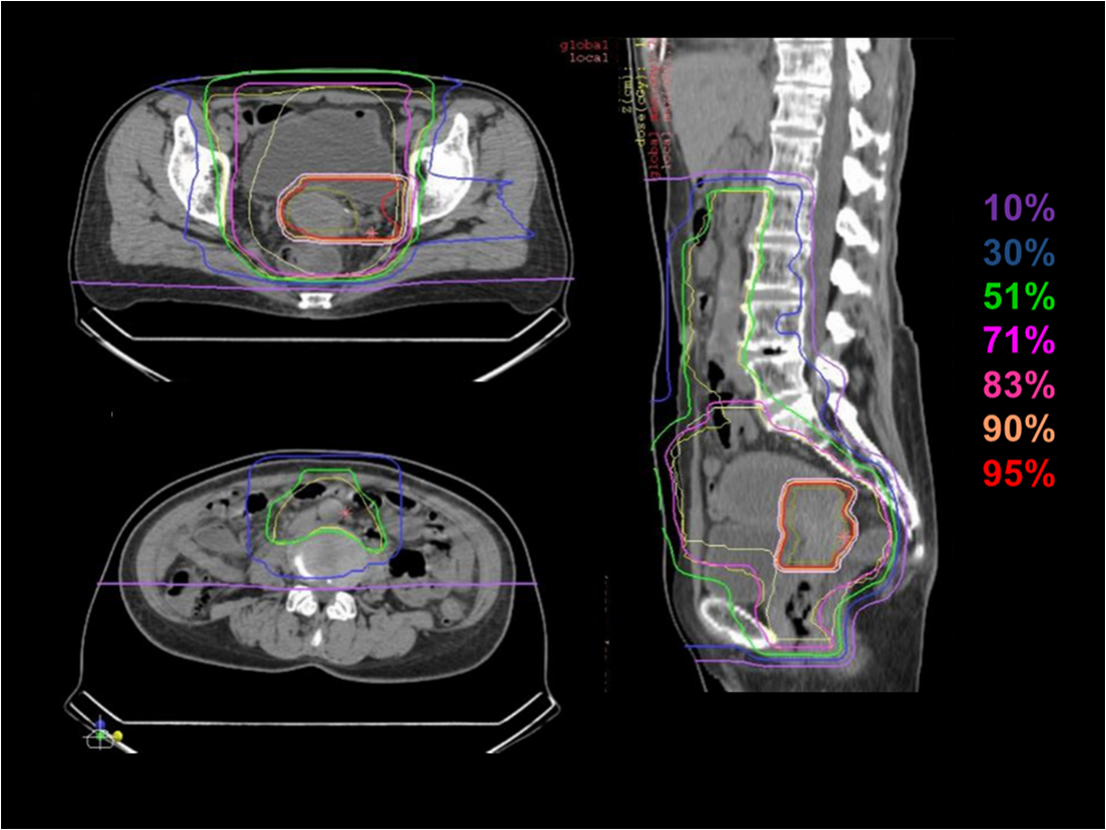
Dose distribution of C-ion RT; Isodose curves of carbon-ion radiotherapy for locally advanced cervical cancer are superimposed on axial and sagittal computed tomography images for the total irradiation plan. Highlighted are 95% (red), 90% (yellow), 83% (orange), 71% (pink), 51% (green), 30% (blue), and 10% (purple) isodose curves.
